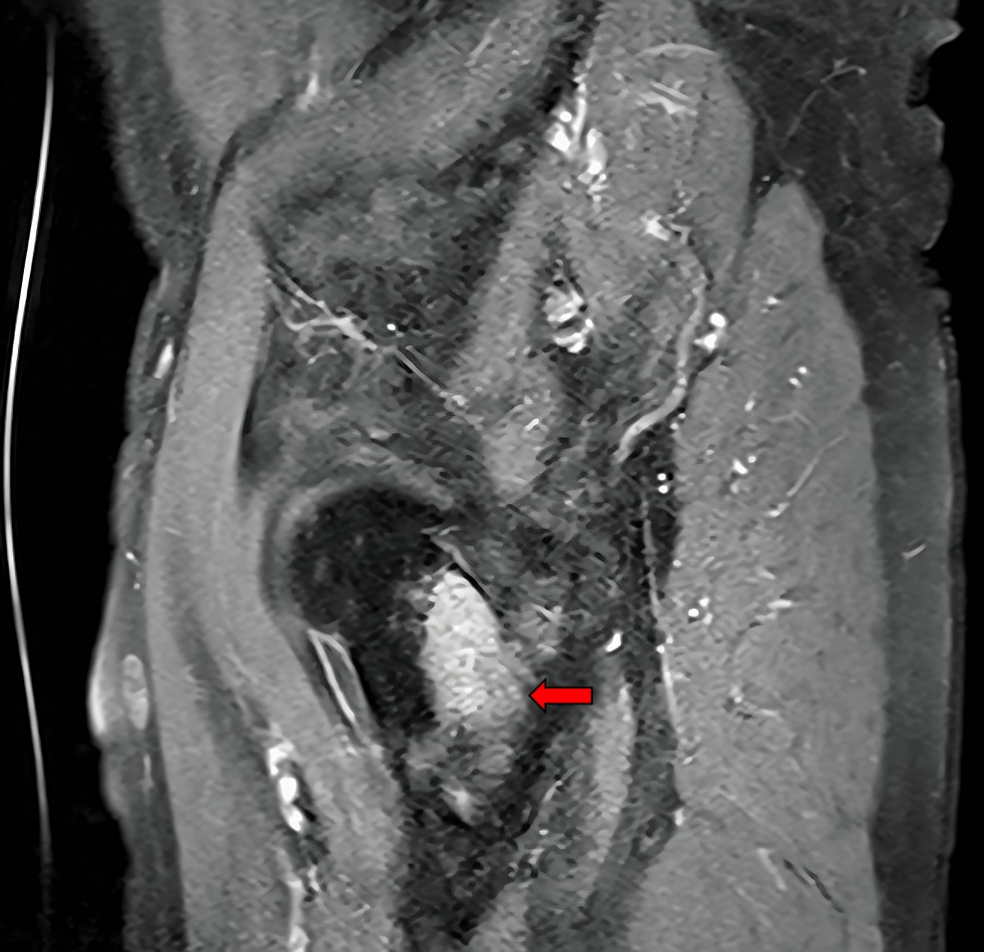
T1-weighted pelvic MRI with contrast, sagittal view An enhancing marrow-based lesion involving the right femoral neck is present with extension into the cortical bone (arrow). The location of this lesion indicates a high risk in this patient for a pathologic fracture with unassisted ambulation.
